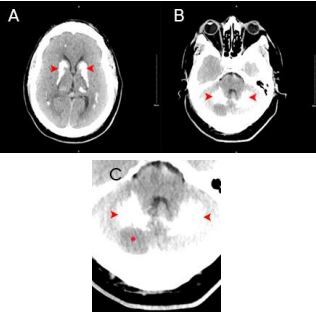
A) Non-contrast computed tomography of the head demonstrates diffuse calcification of the bilateral basal ganglia, thalami, and subcortical white matter of cerebral hemispheres (arrowheads). B) Calcification of the cerebellum bilaterally with area of hypodensity (arrowheads). C) Cerebellum with bilateral calcification (arrowheads) with area of hypodensity noted (asterisk).
